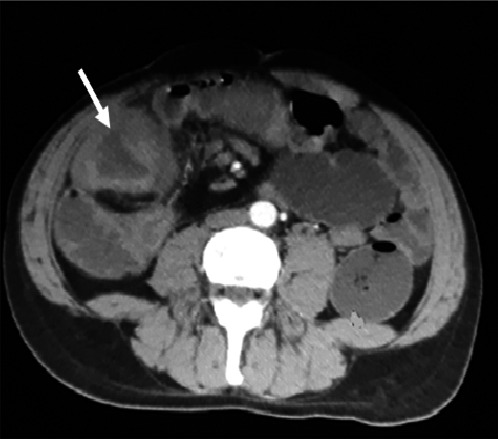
Longitudinal section of abdominal CT showing wall thickening of the right colon (arrow)
